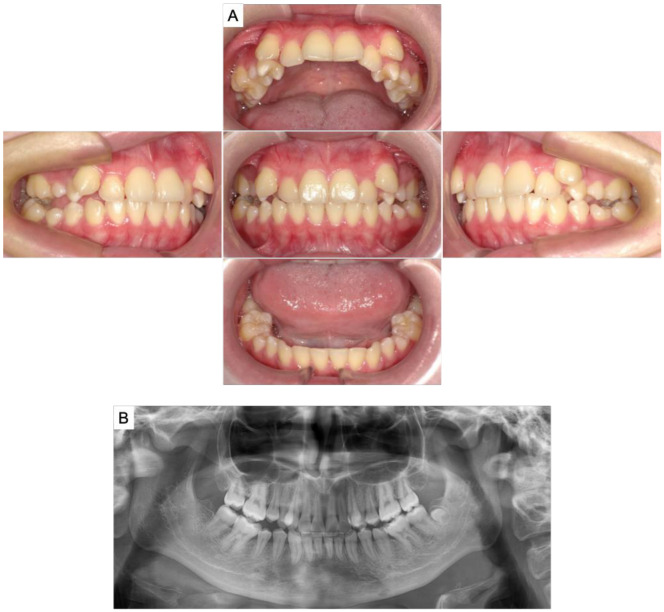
Images at the age of 16 years and 4 months, and no abnormalities were observed in the periodontal tissues. (A) Intraoral photographs. (B) Panoramic radiograph.
